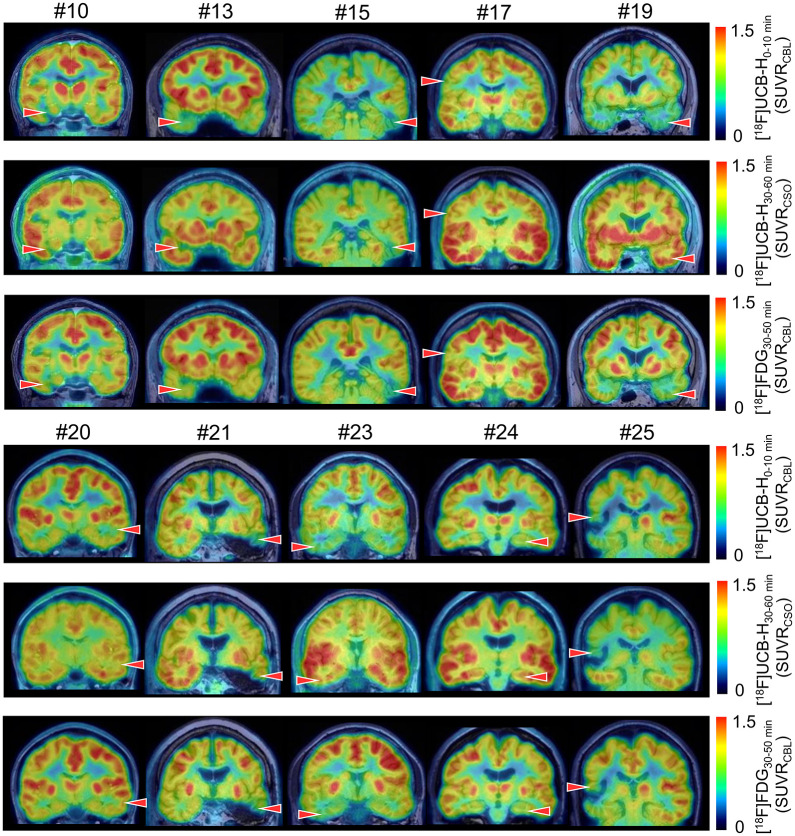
Comparison of early- and late-phase [^18^F]UCB-H images compared with [^18^F]FDG PET. Coronal slices showing early- and late-phase [^18^F]UCB-H PET images alongside [^18^F]FDG PET in 10 representative unblocked patients, overlaid on corresponding T1-weighted MRI. SUVRs were calculated using cerebellar (CBL) scaling for early-phase SV2A PET and [^18^F]FDG PET and centrum semiovale (CSO) scaling for late-phase SV2A PET. Red arrowheads indicate location of epileptogenic lesion identified in each patient.
